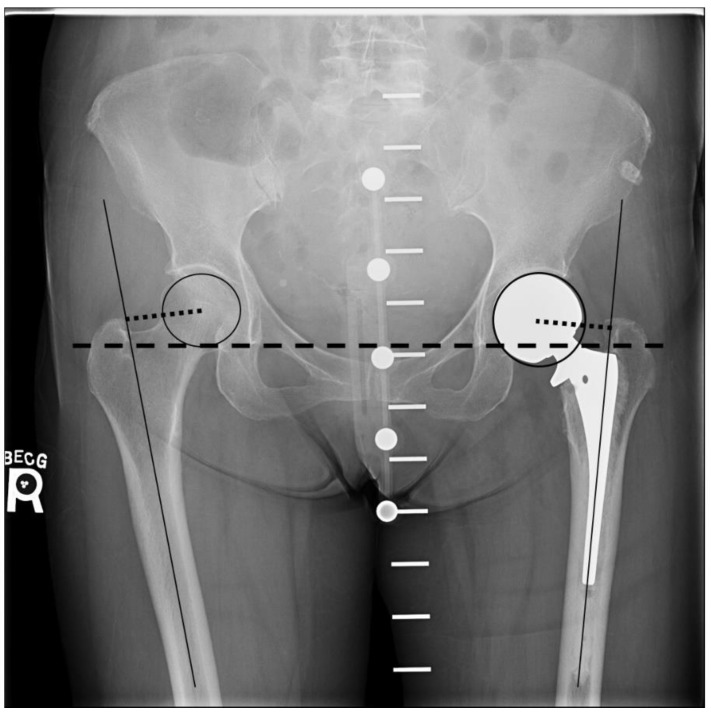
Postoperative AP radiograph of the pelvis with the pelvic calibration device demonstrating a left cemented hemiarthroplasty. The dotted line from the center of the femoral head to the line bisecting the long axis of the femur is the femoral offset. Length discrepancy is determined by measuring the difference in the perpendicular distance from the inter-teardrop line (dashed line) to the superior aspect of the lesser trochanter of each hip.
